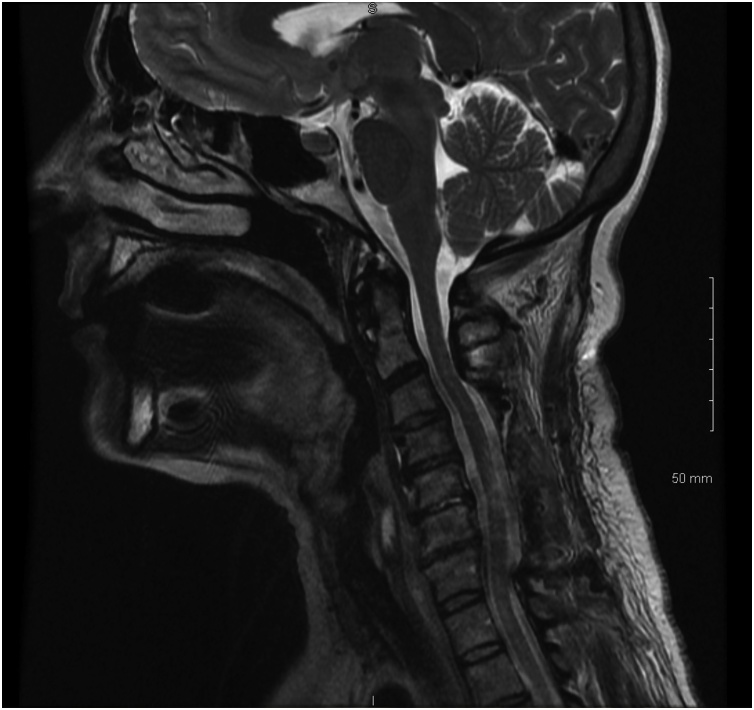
Postoperative MRI Scan of the Cervical Spine.
